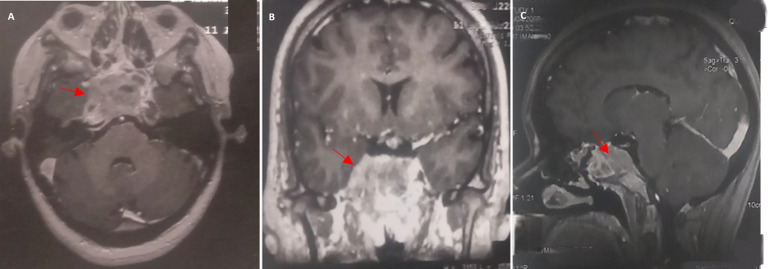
A, B, C) magnetic resonance imaging showing an invasive intra-sellar mass compressing the optic chiasm and completely filling the sphenoid sinus (red arrow)
